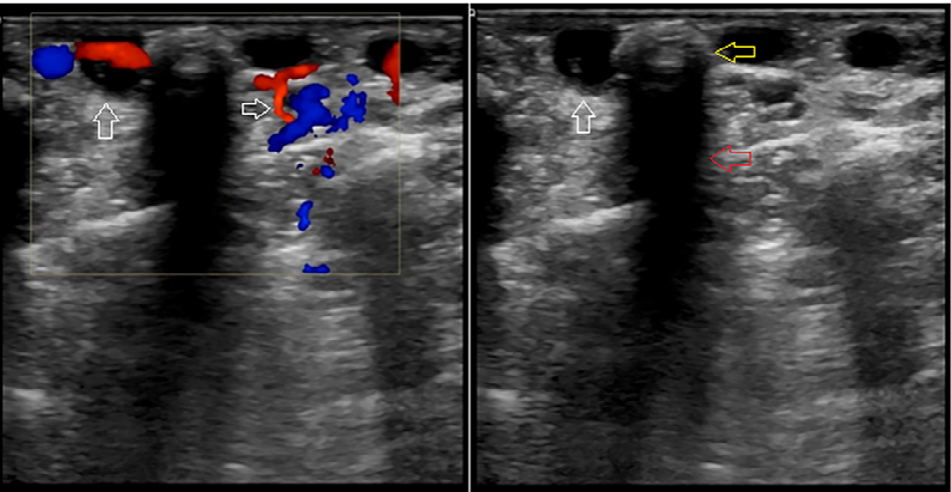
The anechoic, completely compressible structures just below the skin surface had a colour signal on the Doppler ultrasound suggestive of telangiectatic superficial veins (white arrows). Inside these structures were phleboliths (yellow arrow), appearing as echogenic particles with posterior acoustic shadowing (red arrow). (Color version of figure is available online.)
